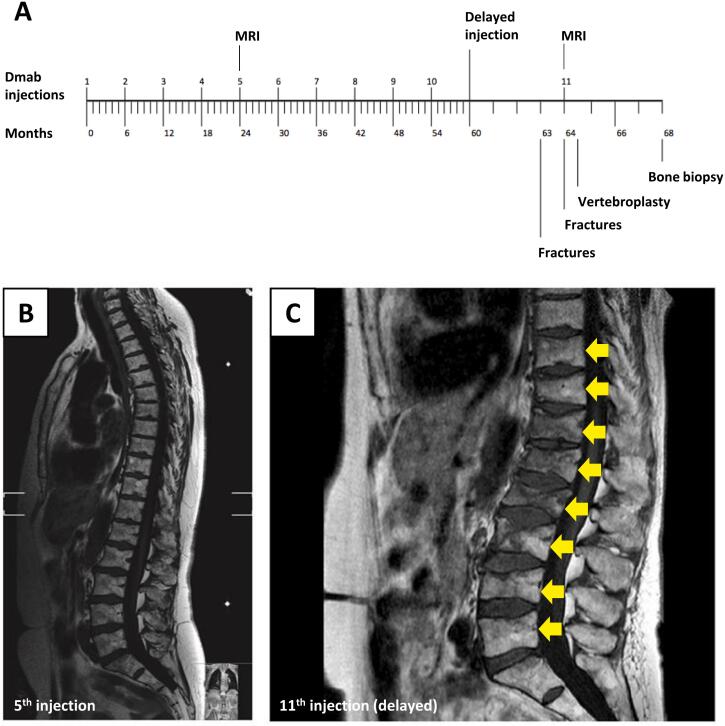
A 64-year-old Caucasian female with osteoporosis experiencing vertebral compression fractures following a delay of the 11th denosumab treatment. A: Timeline showing the patient's regular denosumab injections every 6 months and the 4 months delay of the 11th injection, causing multiple vertebral compression fractures at months 63 and 64. A transiliac bone biopsy was collected at month 68. B-C: Magnetic resonance images (MRI) were obtained at the 5th Denosumab injection (month 48) (B) and month 64, showing vertebral compression fractures (arrows) (C).
